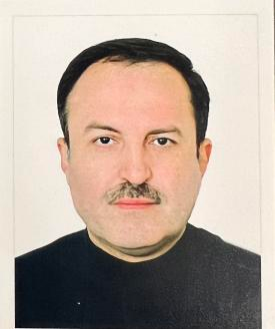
Dr. Abdelaal is a paediatric cardiologist with specialized expertise in advanced echocardiography, including 4D imaging, strain, and myocardial work in congenital heart disease. He trained as a clinical fellow at Great Ormond Street Hospital, London, and is certified by both the European Association of Cardiovascular Imaging (EACVI) and the Association for European Pediatric and Congenital Cardiology (AEPC). He currently practices at the Chest Diseases Hospital, Kuwait, where he supervise clinical care and training in paediatric echocardiography.He is interested in reporting rare cases.
